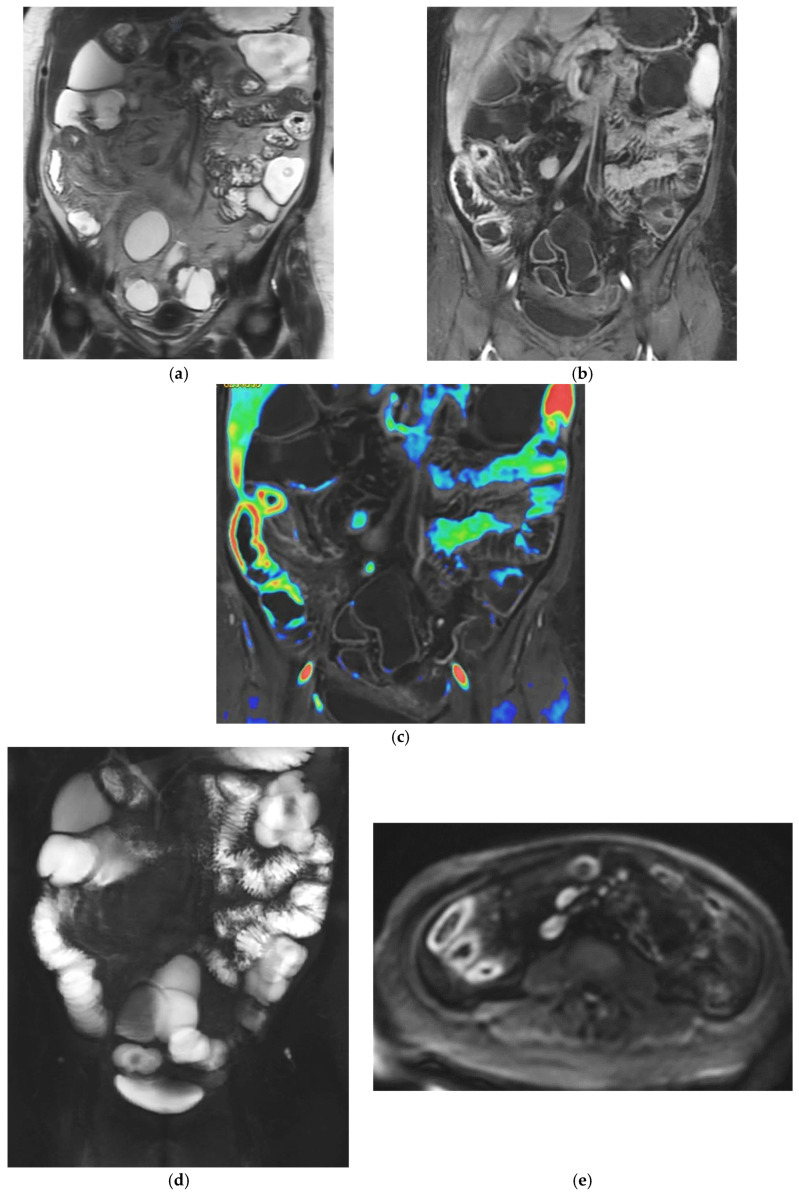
47-year-old female patient with recurrent ileal Crohn’s disease following resection. (a) Coronal T2-weighted image demonstrating recurrent ileal Crohn’s disease at the anastomotic site, characterized by marked bowel wall oedema. (b) Corresponding axial T1-weighted image after gadolinium administration showing intense mural hyperenhancement of the affected ileal loop. (c) Post-processed color-coded map derived from the T1-weighted gadolinium-enhanced sequence, with areas of maximal enhancement highlighted in red. (d) Single-shot fast spin-echo sequence with follow-through–like appearance, clearly depicting the lumen of both normal and pathological small-bowel loops. (e) Axial diffusion-weighted imaging (DWI) showing marked diffusion restriction within the diseased ileal segment.
